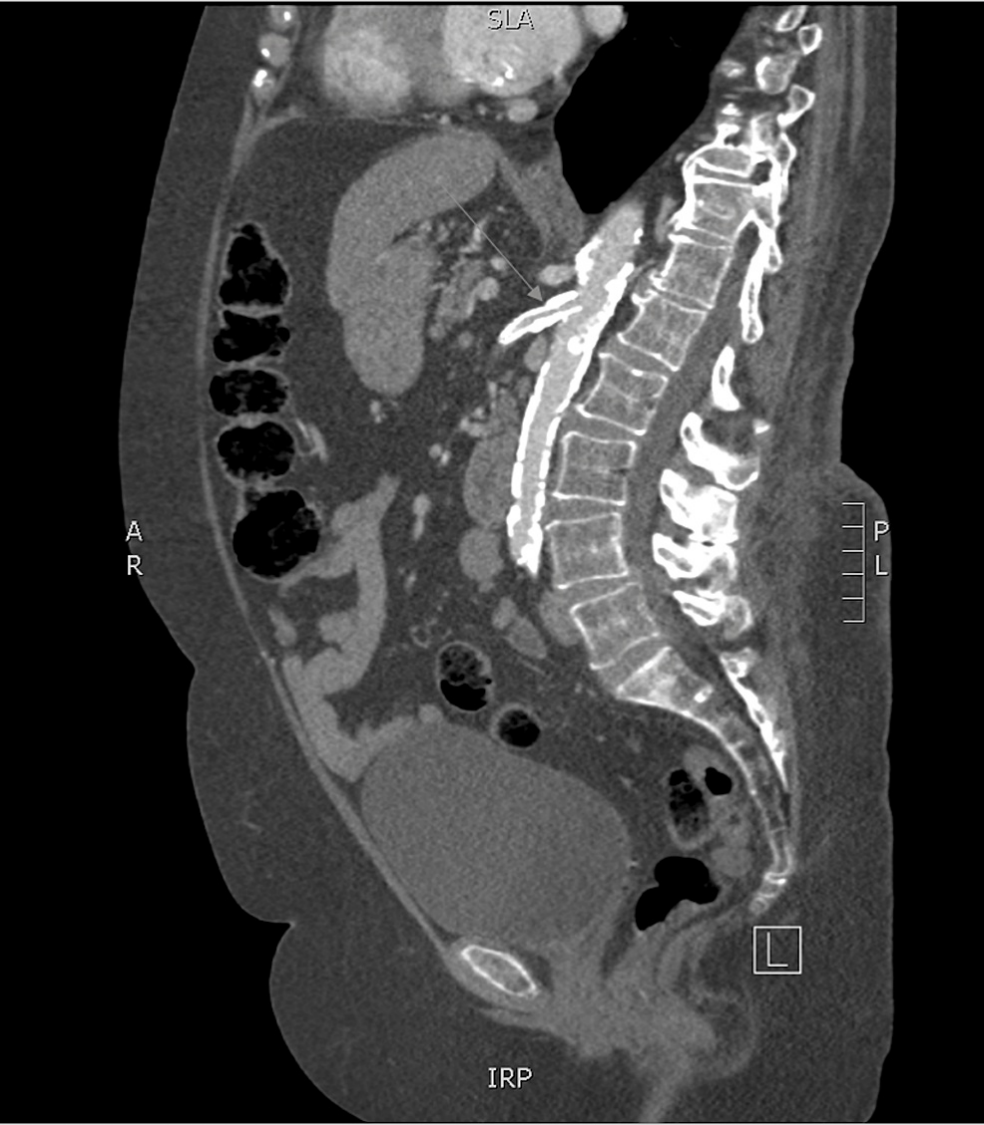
Sagittal CT of the abdomen and pelvis without contrast, demonstrating patency of the superior mesenteric artery after stent placement (arrow). CT, computed tomography
